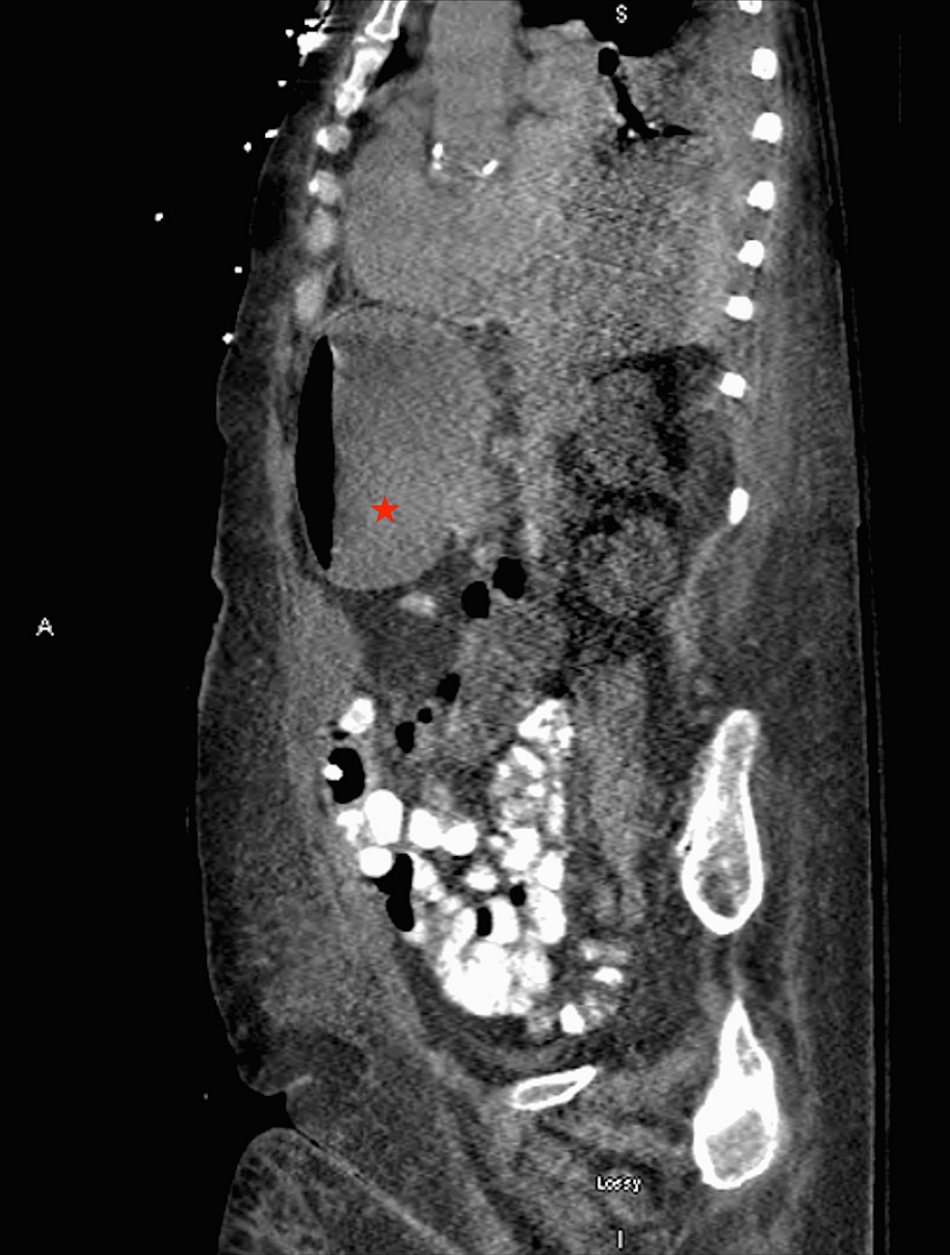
Sagittal abdominal and pelvic CT scan with enteric contrast demonstrating nonspecific gastric and duodenal distention (star) with no evidence of an obstruction given that contrast is seen within the distal bowel
